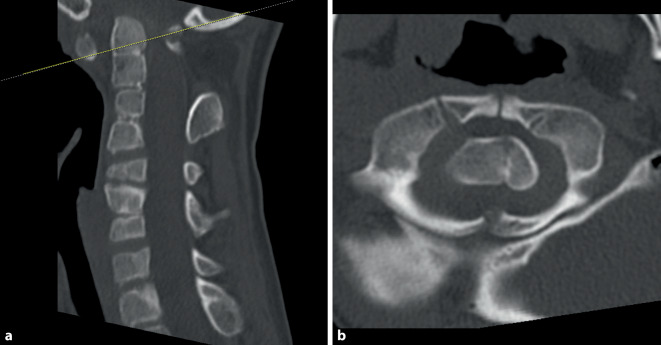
Computed tomography scans in sagittal (a) and axial (b) planes showing the severity of cervical stenosis caused by posterior arch of C1 and C1/C2 instability
